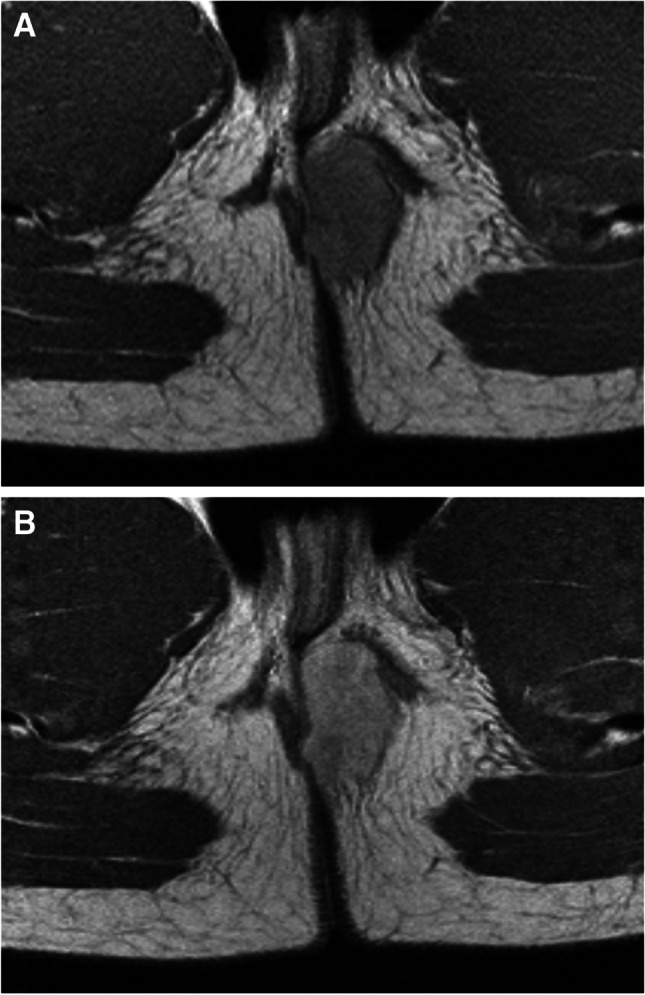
Magnetic resonance image of a primary perianal tumour in a 14-year-old girl (patient 2) with rhabdomyosarcoma. a Axial T1 image shows a solid perianal mass with displacement of and invasion into the internal and external anal sphincters. b Axial T1 image after administration of gadolinium shows homogeneous enhancement
